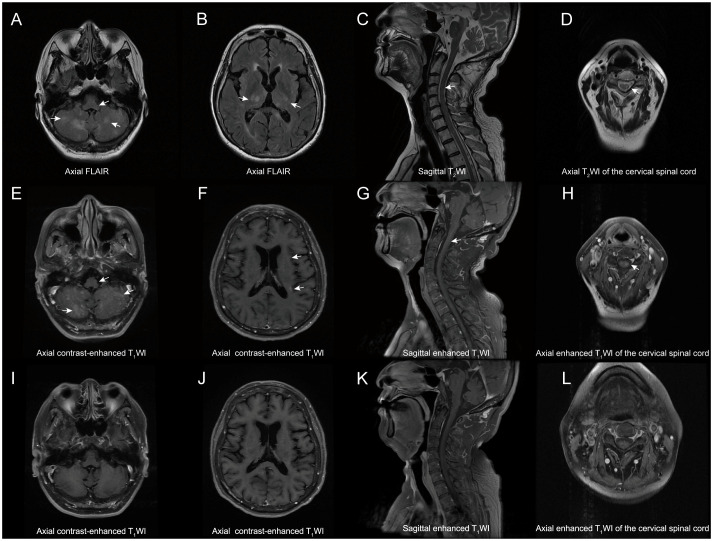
Brain and spinal cord MRI at disease onset (A–H) and post-treatment (I–L). (A, B, E, F) Multiple slightly hyperintense FLAIR signals with enhancement are present in the brainstem, cerebellar gray and white matter (A, E), the bilateral subcortical and deep cerebral white matter, thalami (B, F). (C, D, G, H) Multiple small patchy slightly hyperintense T_2_WI signals with enhancement in the cervical spinal cord parenchyma. (I, J) Reduction in the number and size of lesions in the bilateral cerebral hemispheres, brainstem, and cerebellar hemispheres. (K, L) No abnormal signal or abnormally enhancing lesions are seen in the cervical spinal cord. MRI, magnetic resonance imaging; T_1_WI, T_1_-weighted imaging; T_2_WI, T_2_-weighted imaging; FLAIR, fluid-attenuated inversion recovery.
